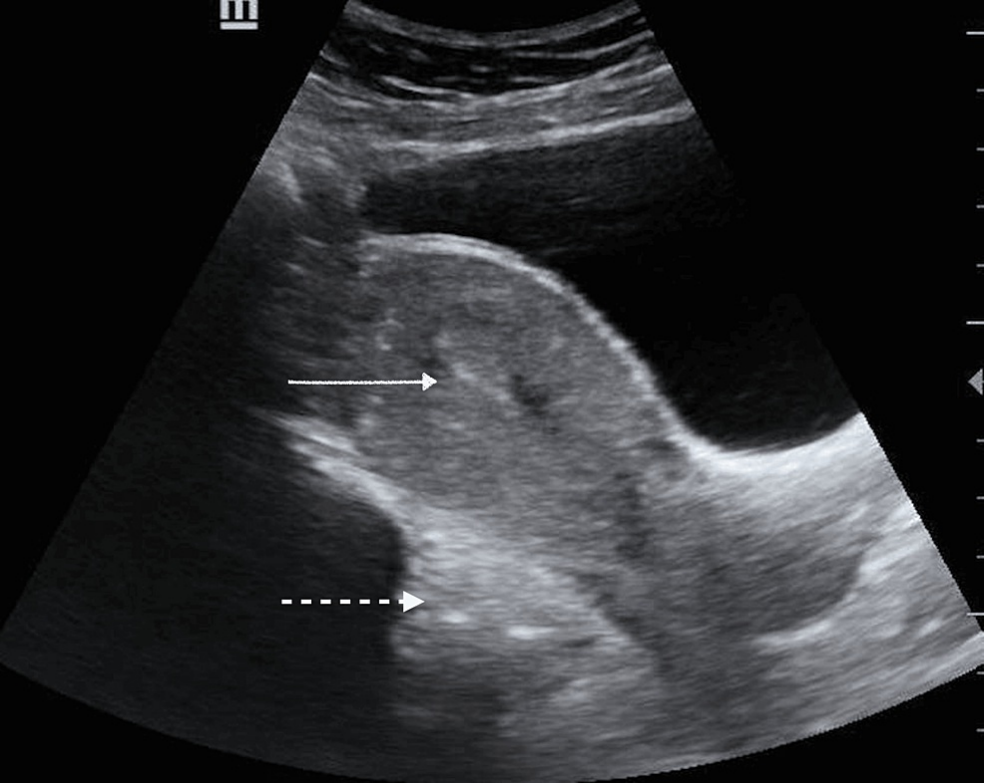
Transvaginal ultrasound image Thickened endometrium with no intrauterine gestational sac (solid white arrow) with hyperechoic collection in the pouch of Douglas (dotted white arrow)
